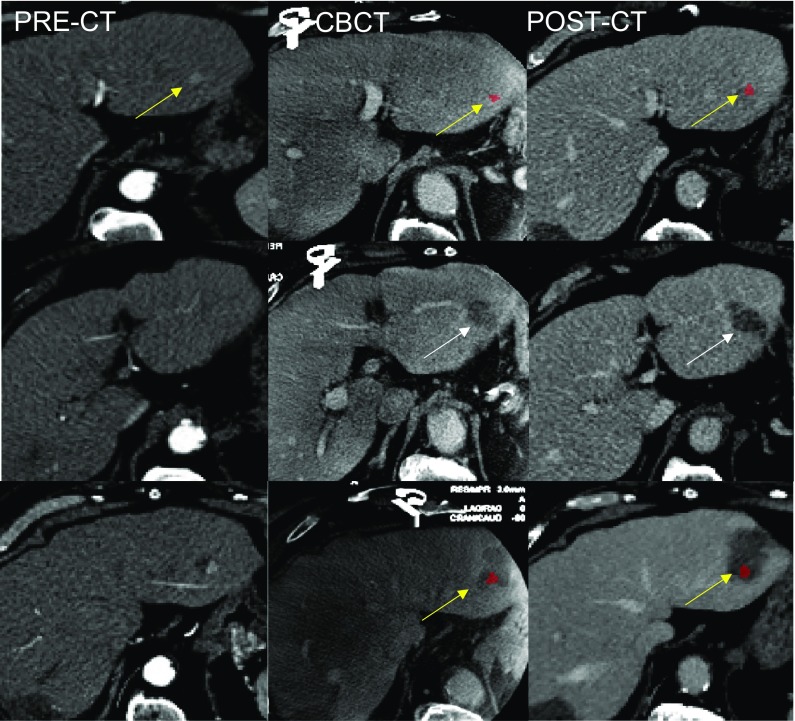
CBCT demonstration of missed target. Comparison among pre-ablation CT (PRE-CT), intraprocedural registered CBCT, and 24 h post-ablation CT (post-CT) (second HCC of patient #2). After the first treatment, both CBCT and post-CT show that the HCC (colored in red and indicated by yellow arrows) is entirely located outside the volume of ablation (missed target) (top row). Most notably, the ablated area (indicated by the white arrows of the second row) is located caudally with respect to the actual target position (yellow arrows of the first row) both on CBCT and post-ablation CT. In the bottom row, the successful retreatment is shown, with the ablated area (yellow arrows) fully covering the target (red dot)
